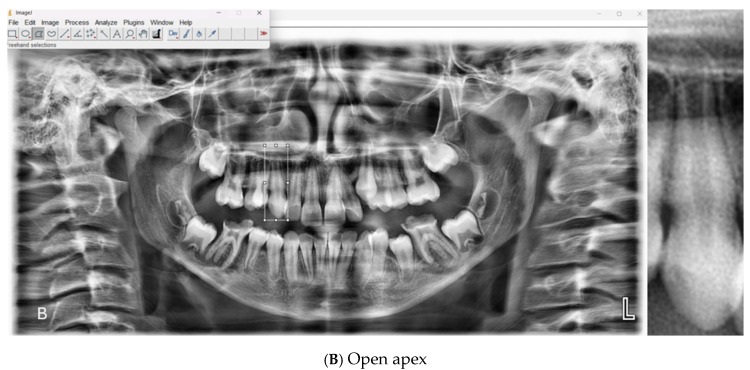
Representative panoramic radiographs and cropped regions of interest (ROIs) showing (A) closed apex and (B) open apex teeth.
